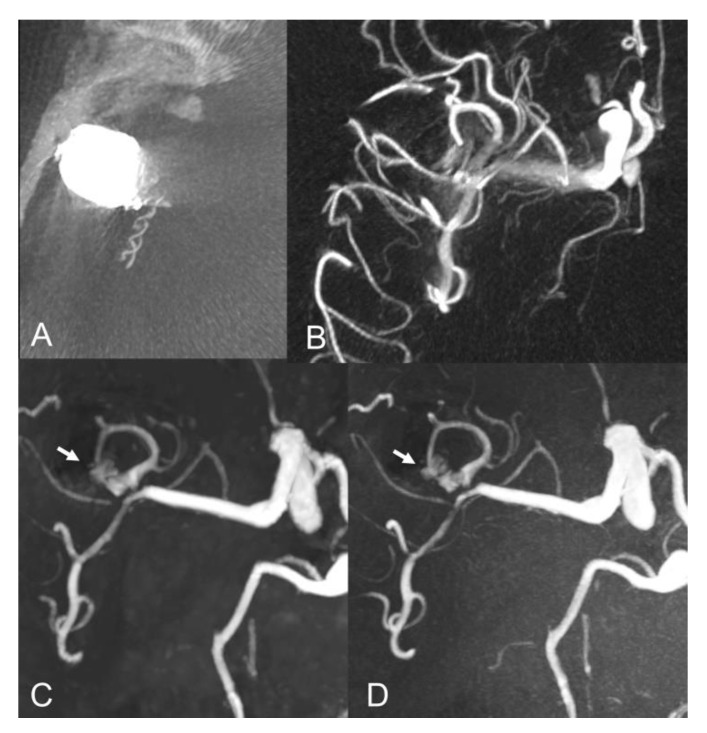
Baseline axial CT image (A) and vaso-CT (B) after stent-assisted coiling of a middle cerebral artery aneurysm. Follow-up axial MIP images of CS TOF (C) and PI TOF (D) showing residual flow (arrows) between the coils (class IIIb).
